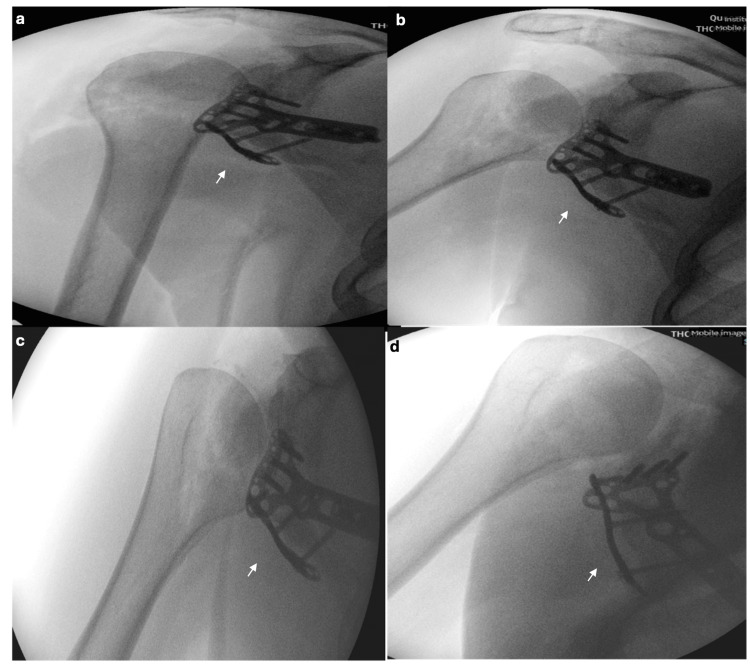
Intra-operative X-rays taken with an image intensifier machine. a. Anterior-posterior X-ray with the arm adducted. b. Anterior-posterior X-ray with the arm adducted. c. Lateral X-ray with the arm in adduction. d. Lateral X-ray with the arm in abduction. Figures 2a-2d show that the glenoid fracture has been successfully reduced and internally fixated with the use of a distal radius plate and screws. The fixation remained stable on anterior-posterior and lateral views with the abduction-adduction movement of the arm (as indicated by arrows).
